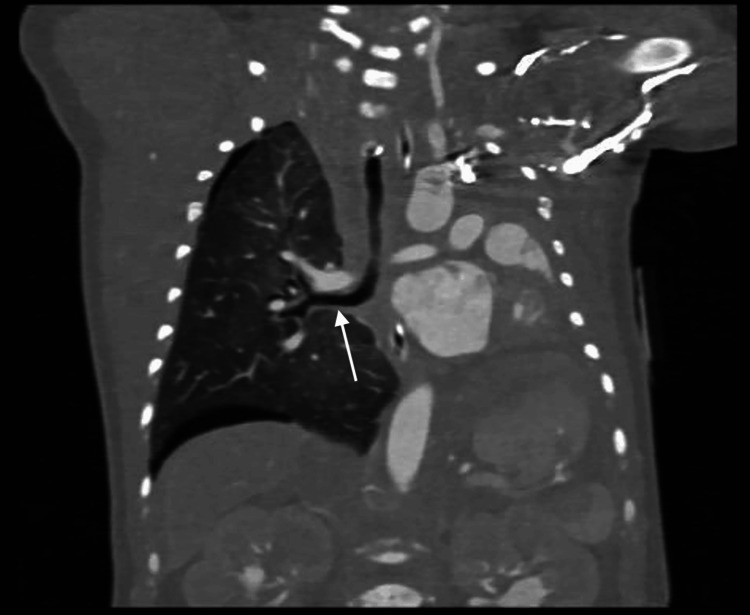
Coronal computed tomography scan demonstrating left lung agenesis and leftward mediastinal shift The left main bronchus is absent, and only the right main bronchus is visualized (white arrow).
